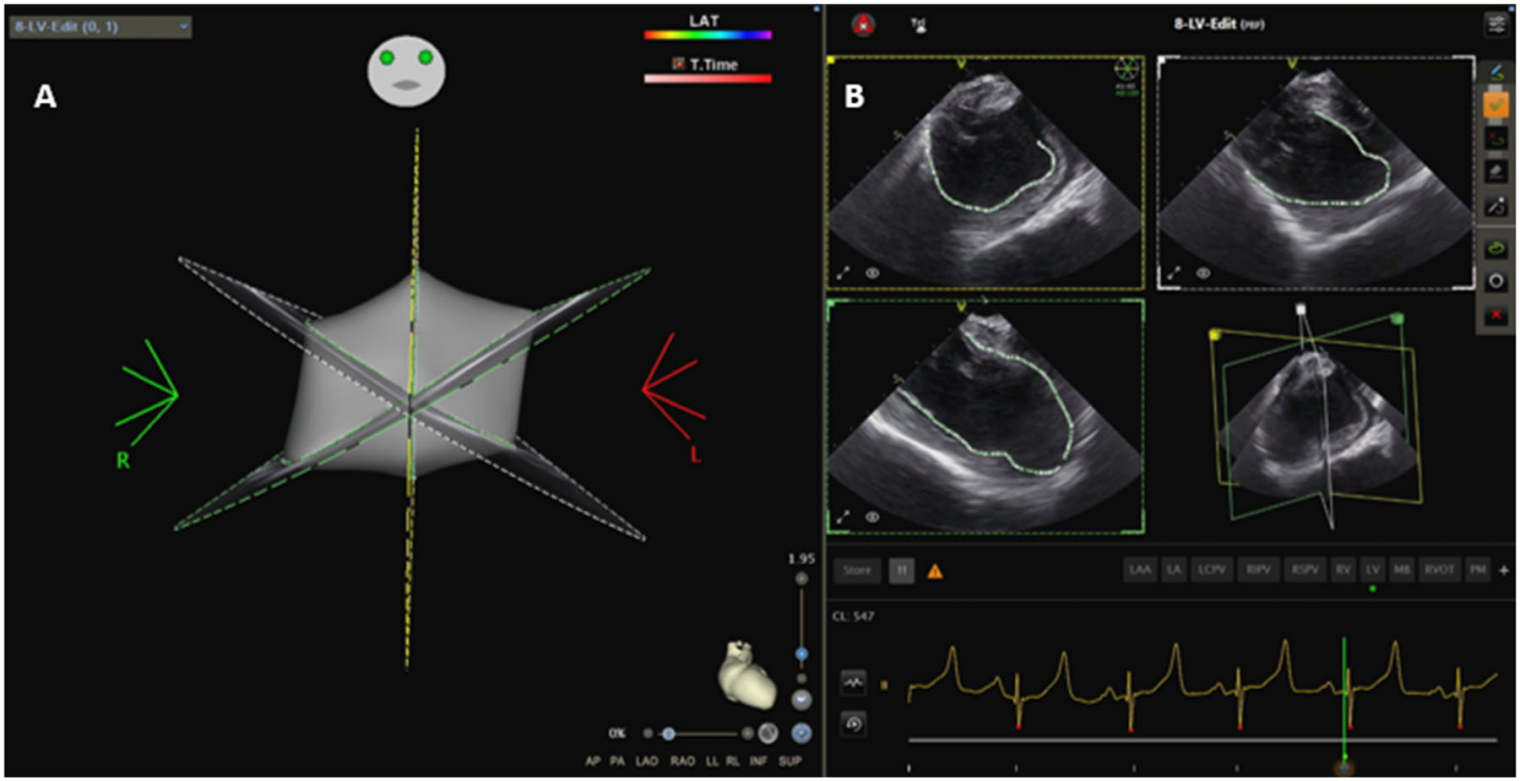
4D ICE creation of a left ventricular shell using CARTOSOUND and sound to FAM using multiplane acquisition (A). Ultrasound views of multiple simultaneously acquired planes allowing for contour creation in multiple planes without catheter manipulation (B).
